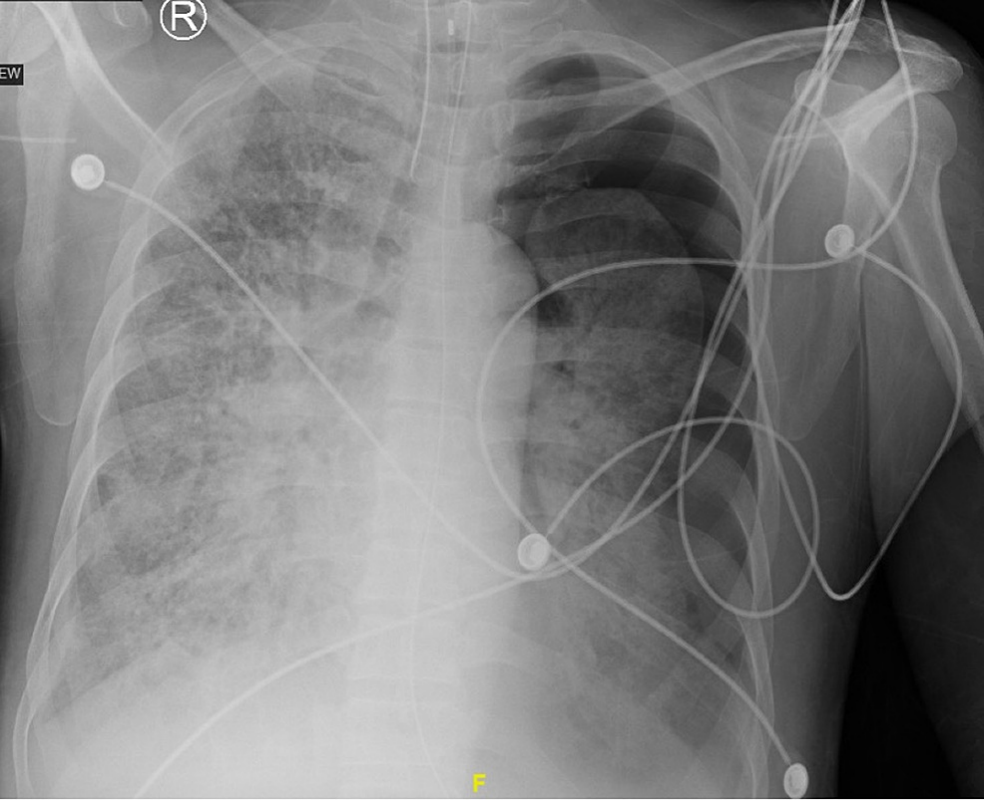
Chest radiograph showing a large left-sided apical pneumothorax with collapse of the left lung
